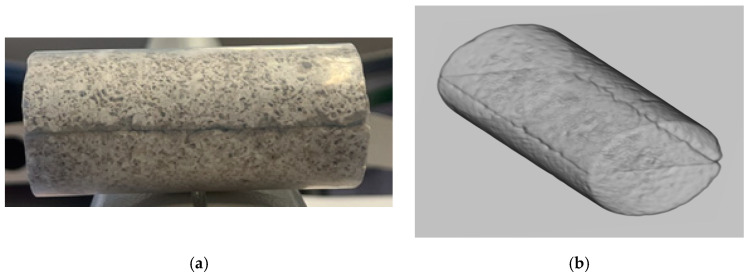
Fractured core sample images: (a) prepared core sample; (b) 3D CT scan image.
